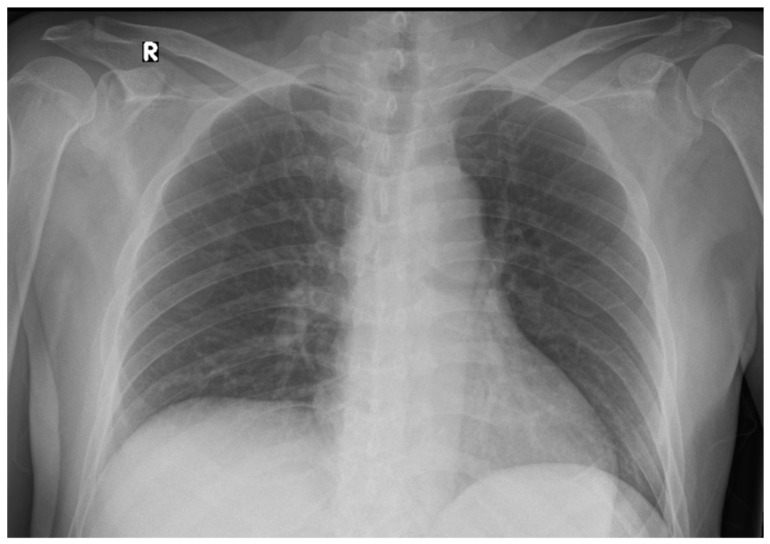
Chest X-ray in the emergency department showed unremarkable findings.
